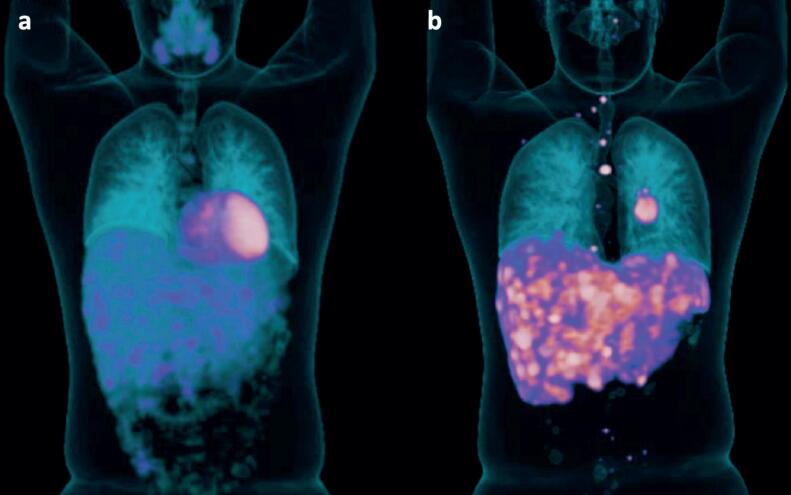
^18^F-FDG PET CT in coronal view (a) and ^68^Ga-DOTATOC PET CT in coronal view (b). ^18^F-FDG PET CT: discrete increase in glycolytic metabolism in the ILL (SUVmax = 5.7 / 5.1×3.7 cm), left lobe hilum (SUVmax = 3.0), hepatic hypovascular lesions (SUVmax = 5.6 / 2.2 cm in segment IVA and SUVmax = 6.6 in segment V), spine (SUVmax = 5.2 in D5), sacrum (SUVmax = 3.2) and pelvis (SUVmax = 3.3 left iliac). ^68^Ga-DOTATOC PET CT: significant increase of somatostatin receptor expression in the ILL (SUVmax = 22.7/5.1×3.7 cm), left lobe hilum (SUVmax = 17.3), hepatic hypovascular lesions (SUVmax = 25.1 in segment IVA and SUVmax = 37.3 in segment V), and multiple bone lesions, predominantly sclerotic, in the spine (SUVmax = 45.0 in D5), left sacrum (SUVmax = 3.2) and left pelvis (SUVmax = 12.6).
